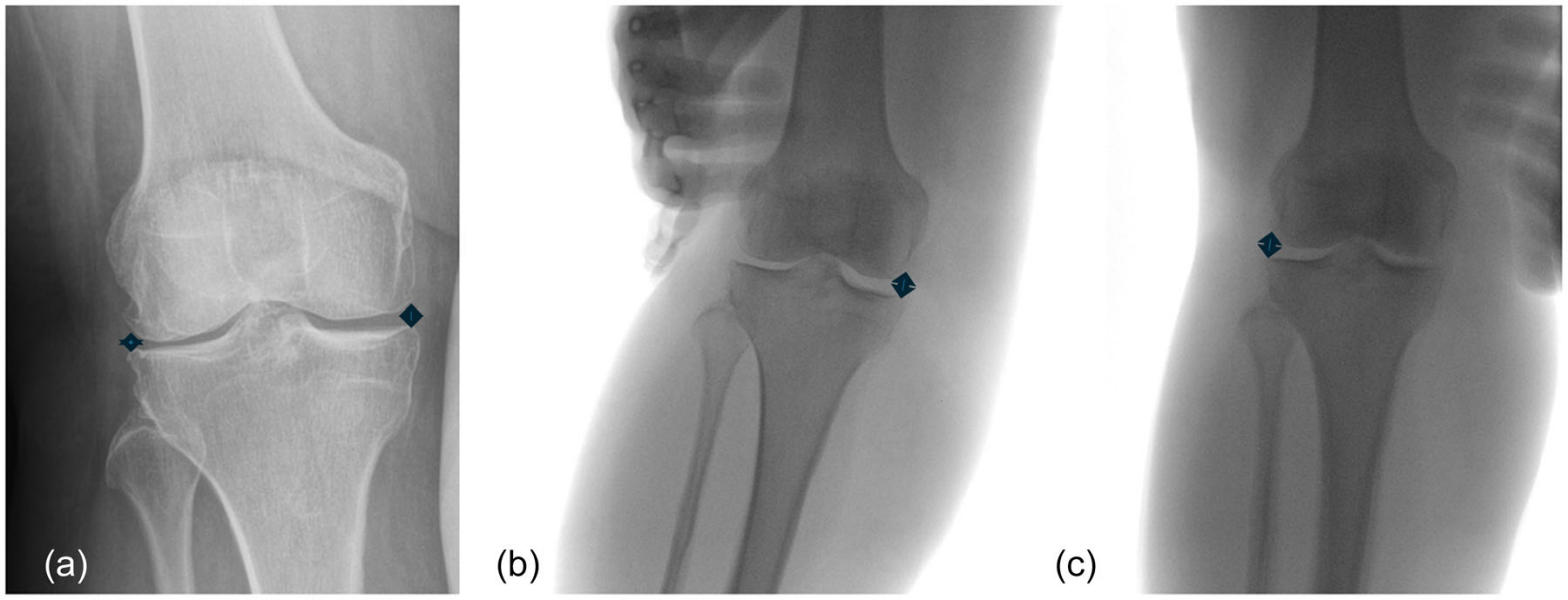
Stress radiographs. (a) Neutral view. (b) Forced valgus for medial compartment. (c) Forced varus for lateral compartment.
